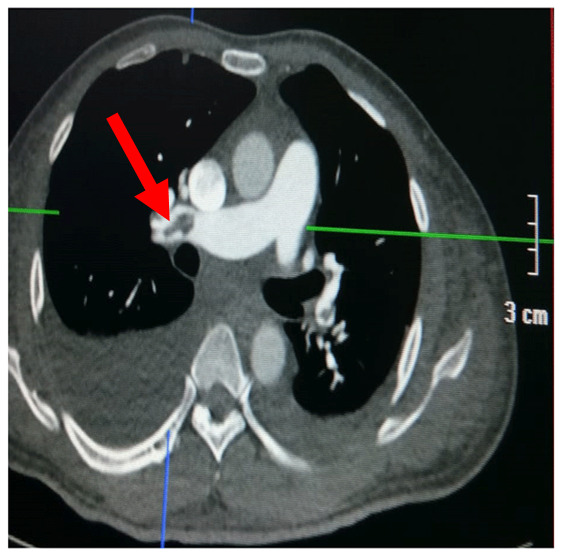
Chest CT scan of the patient. Red arrow showing the location of the thrombus.
